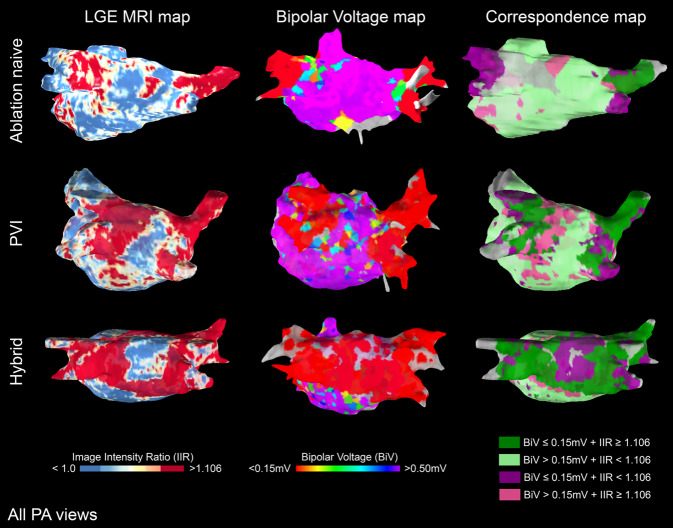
Assessment of atrial structural substrate and atrial fibrillation ablation scars: late gadolinium enhancement (*LGE*) magnetic resonance imaging (*MRI*) maps (*left*) and bipolar electroanatomical voltage maps (*right*) of a patient who was ablation-naïve (*top*), a patient who had undergone intracardiac pulmonary vein isolation (*PVI*) (radiofrequency catheter ablation), and a patient who had undergone epicardial hybrid atrial fibrillation ablation (radiofrequency ablation clamp). In both technologies, *red* represents scar regions. (For color codes of the image intensity ratio and bipolar voltages, see below the figure; all posteroanterior views)
